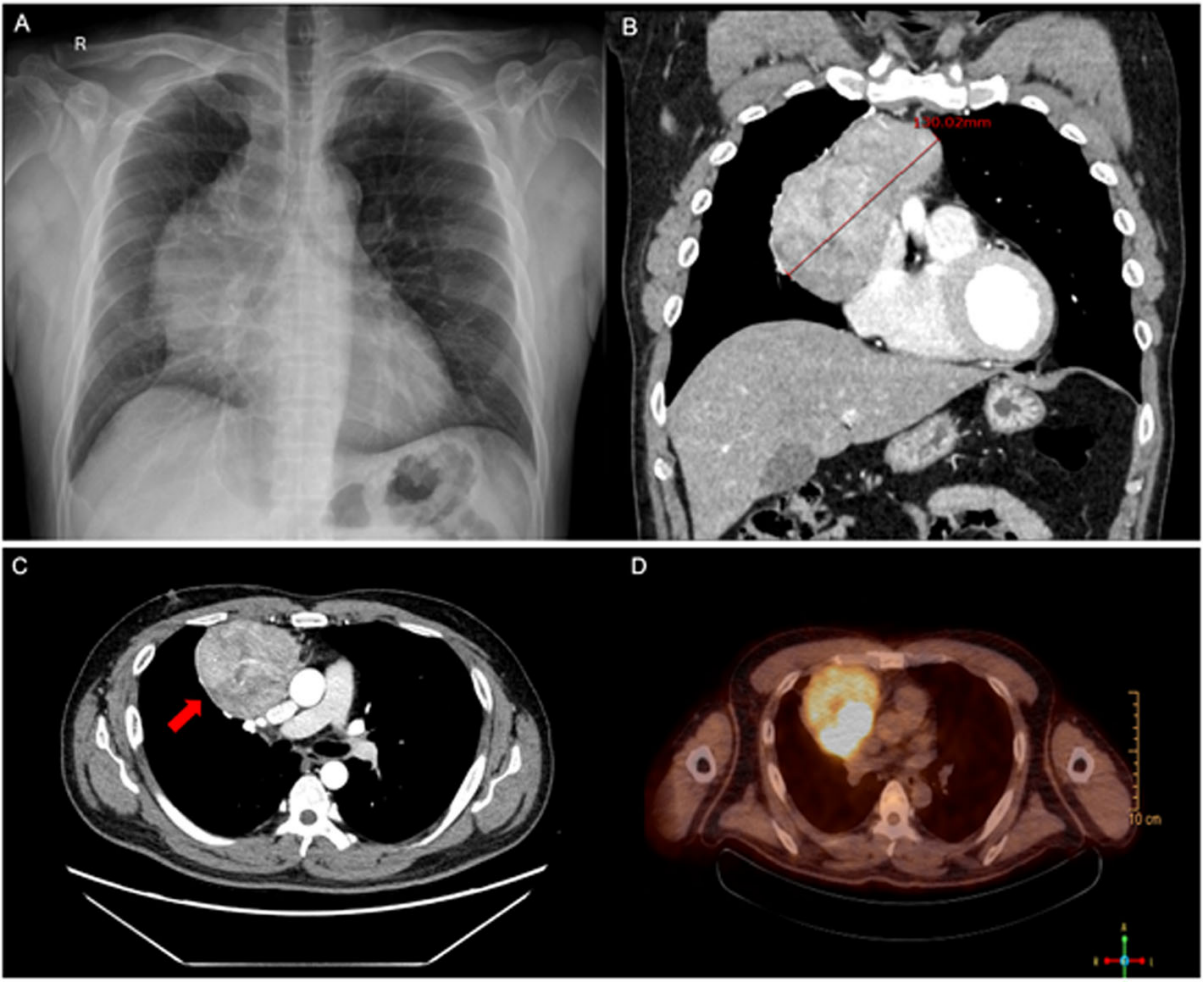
Images of the mediastinal mass. a Chest X-ray showing a lobulated right cardiomediastinal contour. b Contrast enhanced computed tomography (CT) showing a 13-cm-sized mediastinal mass in the sagittal plane (red arrow). c CT showing an anterior mediastinal mass invading the superior vena cava and pericardium (red arrow). d Fluorodeoxyglucose (FDG) positron emission tomography showing intense FDG uptake within the mass, with an SUVmax of 10.1
